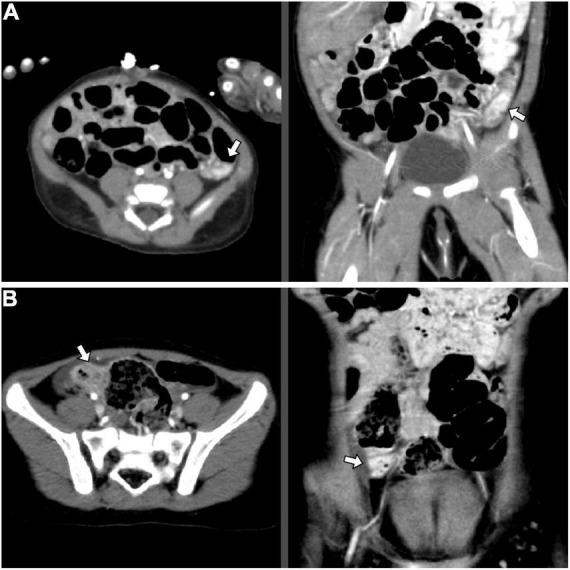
Abdominal CT angiography images indicating corresponding locations of the AD, axial (left) and coronal (right) view: (A) Case 2: Wall enhancement of focal segments at the left lower abdomen, suspected of hemangioma or hypervascular lesions in the descending colon (white arrow); (B) Case 3: Focal increased wall enhancement at the ascending colon (white arrow). AD, angiodysplasia; CT, computed tomography.
